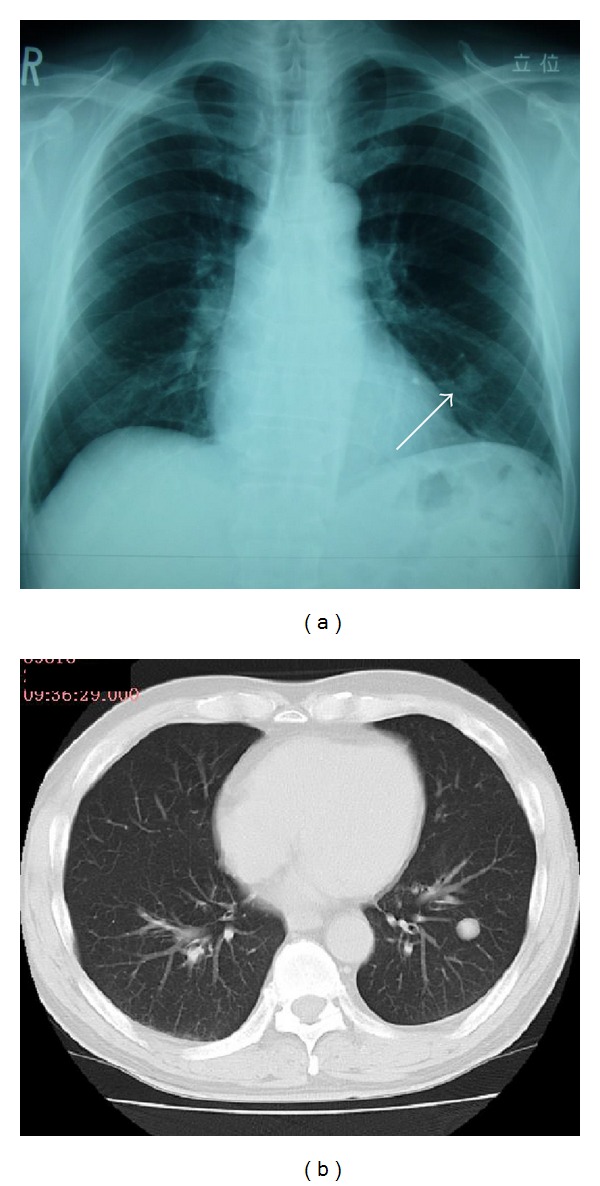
Chest X-ray and CT findings on admission. (a) Simple chest X-ray indicated a smooth, well-circumscribed nodule in the left lower lung field (white arrow). (b) Simple chest computed tomography revealed a nodule measuring 17 mm in diameter and having a well-defined border in S9 of the left lung.
